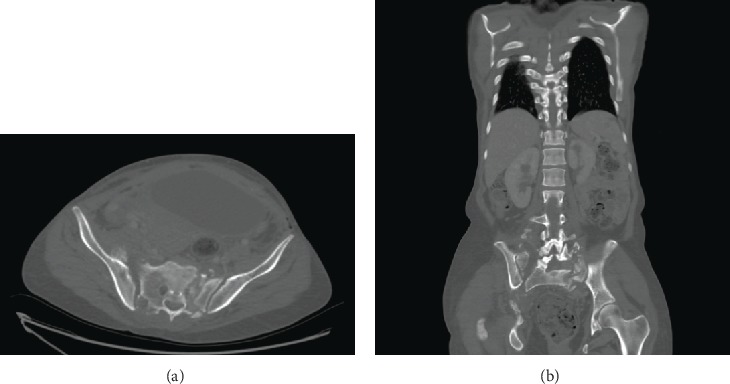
(a, b) CT scan of the pelvis and spine in axial (a) and coronal (b) cuts, respectively, showing spinopelvic dissociation with severe osseous destruction extending from the ala into S1 and S2.
